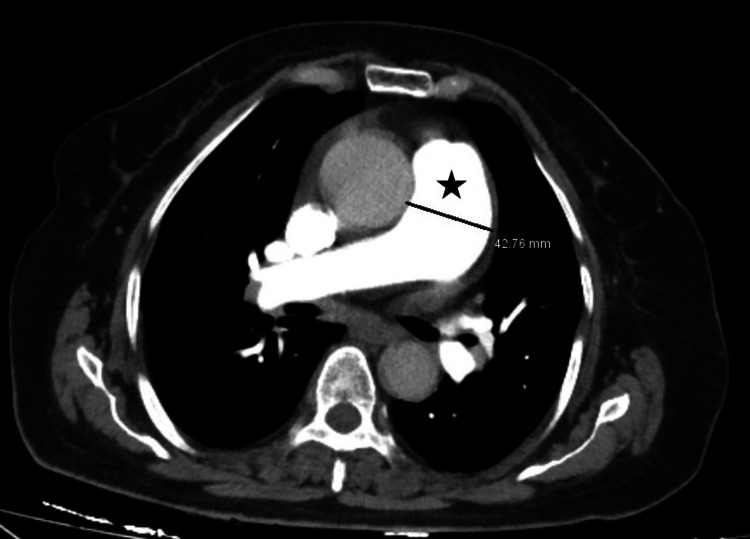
Axial CT measuring the diameter of the main pulmonary artery (marked by star) in mediastinal window setting
